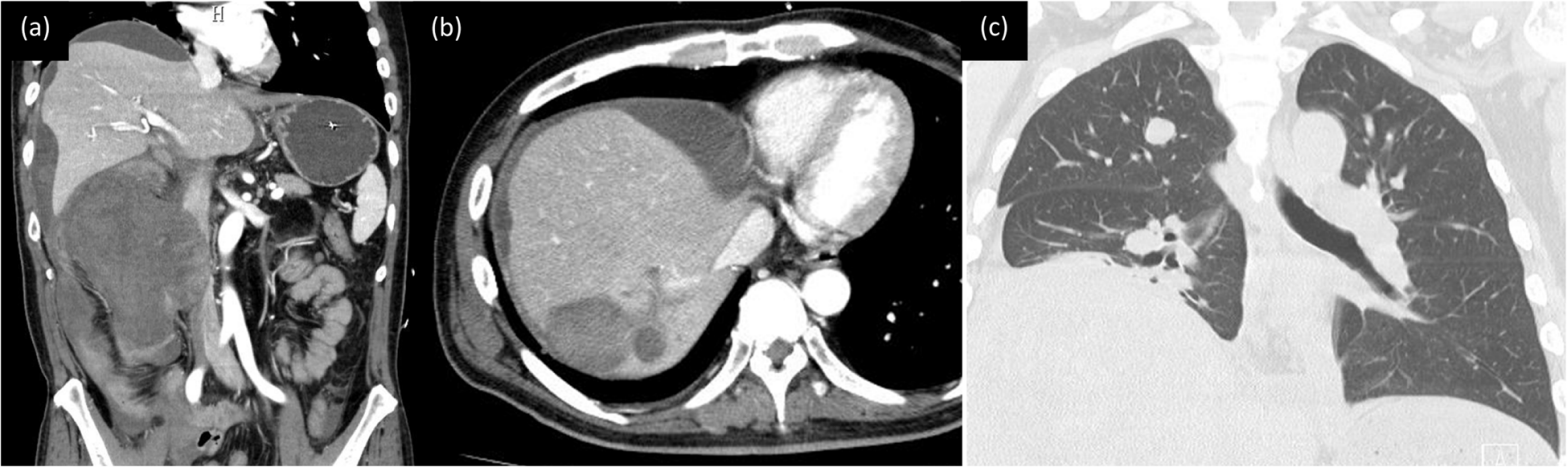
a-c Computed tomography scan shows a greater than 16 cm recurrent tumor with distant metastases in the posterior segment of the liver and the lingula of the lung
